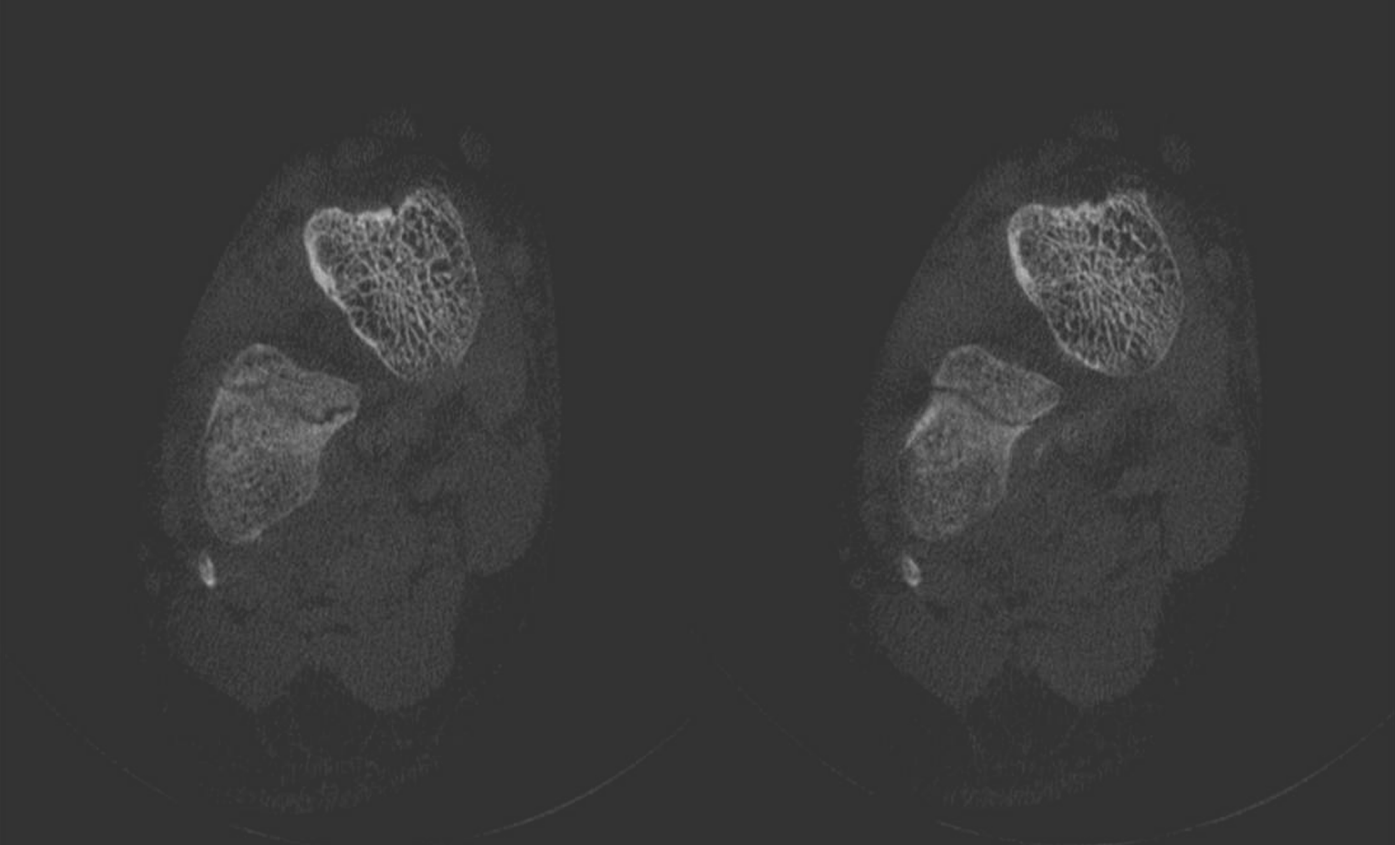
Scans of the calcaneus with a discrete fracture without dislocation of the bone: CBCT taken 6 weeks later at a follow-up examination
